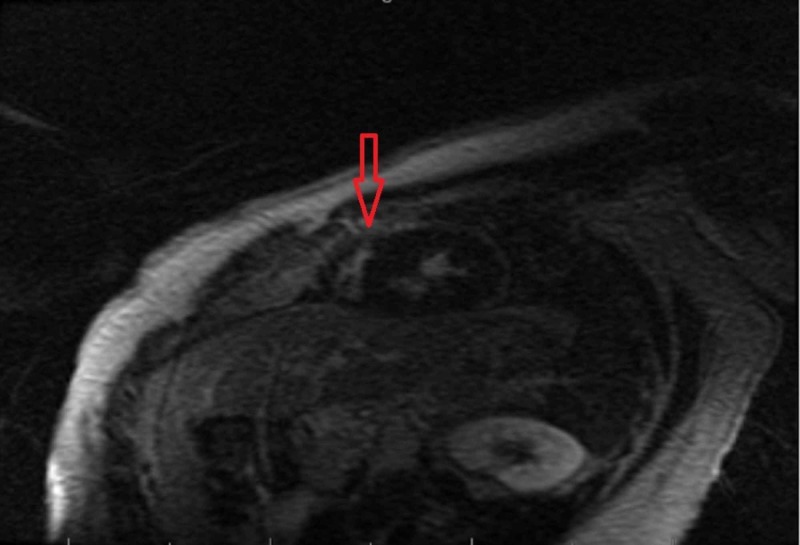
Cardiac magnetic resonance imaging showed thickening of the posterior septum with meso-myocardial delayed enhancement (red arrow)
